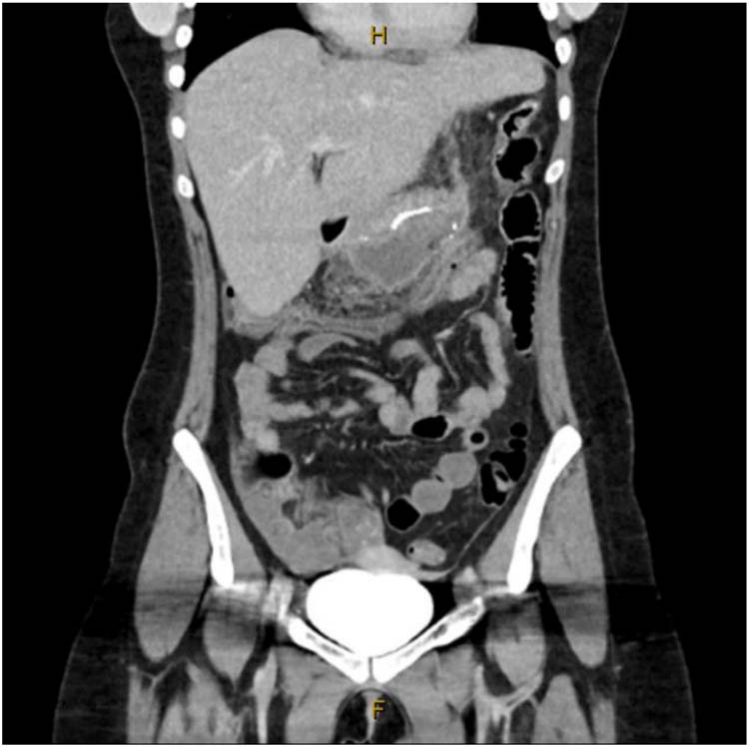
Coronal CT image showing staple line collection at time of presentation. The image shows a 14 × 7 × 5 cm^3^ collection adjacent to the sleeve gastrectomy staple line.
